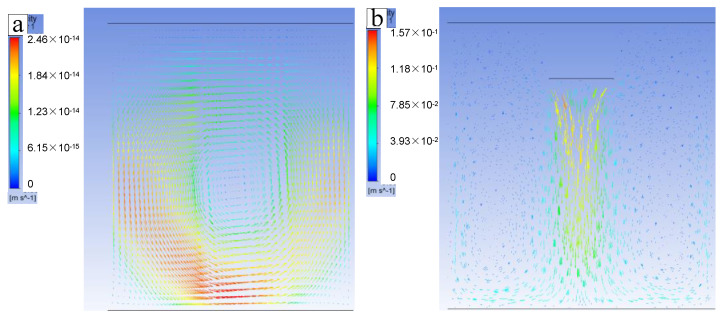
Flow patterns and velocity of the melts under (a) no ultrasonication and (b) ultrasonication.
